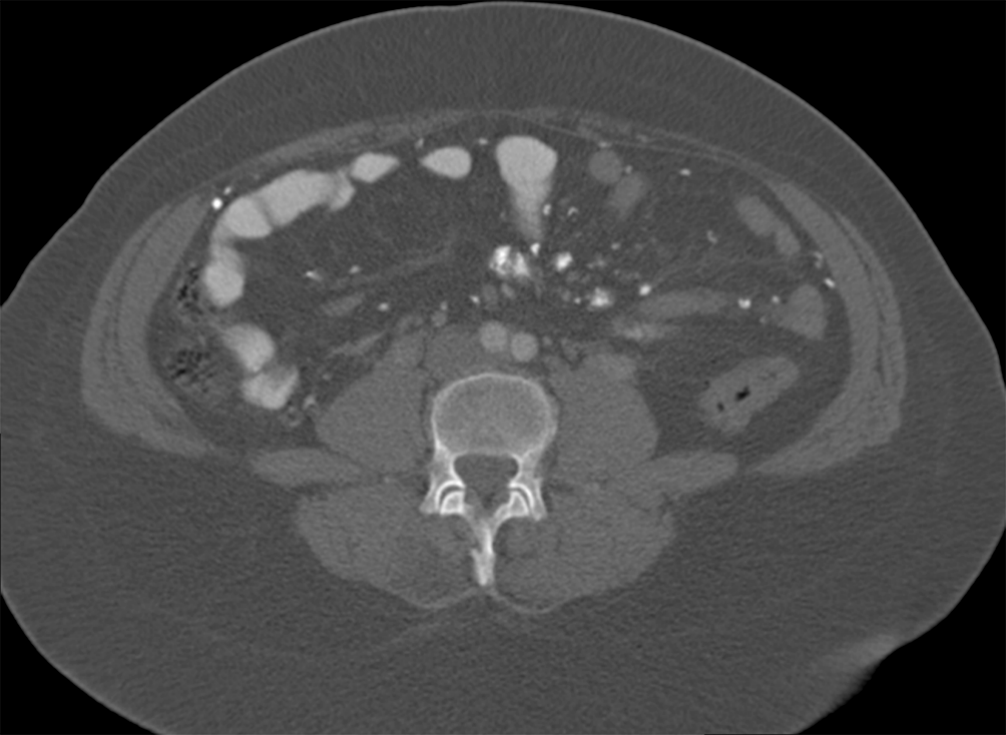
Unchanged calcifications in the peritoneum and mesentery on repeat CT.
